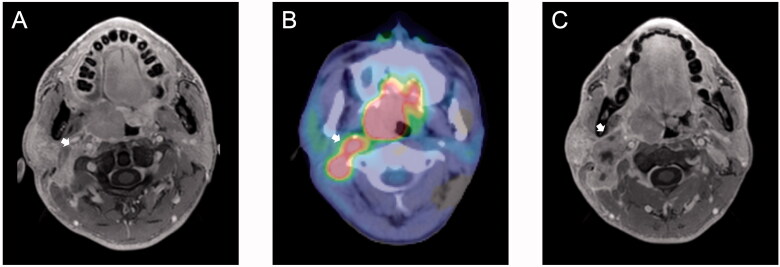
Oropharyngeal carcinoma with bilateral multiple cervical lymph nodes (LNs) and ipsilateral retro-styloid lymph node metastasis (RSLNM). (A) Contrast-enhanced fat-saturated eTHRIVE and (B) [^18^F]-fluoro-2-deoxy-d-glucose-positron emission tomography/computed tomography fused images show the presence of RSLNM (arrow; longest diameter = 14 mm, SUV_max_=4.1). (C) Contrast-enhanced fat-saturated eTHRIVE images. The longest diameter of LN in the upper limit of ipsilateral level II was 27 mm (arrow).
